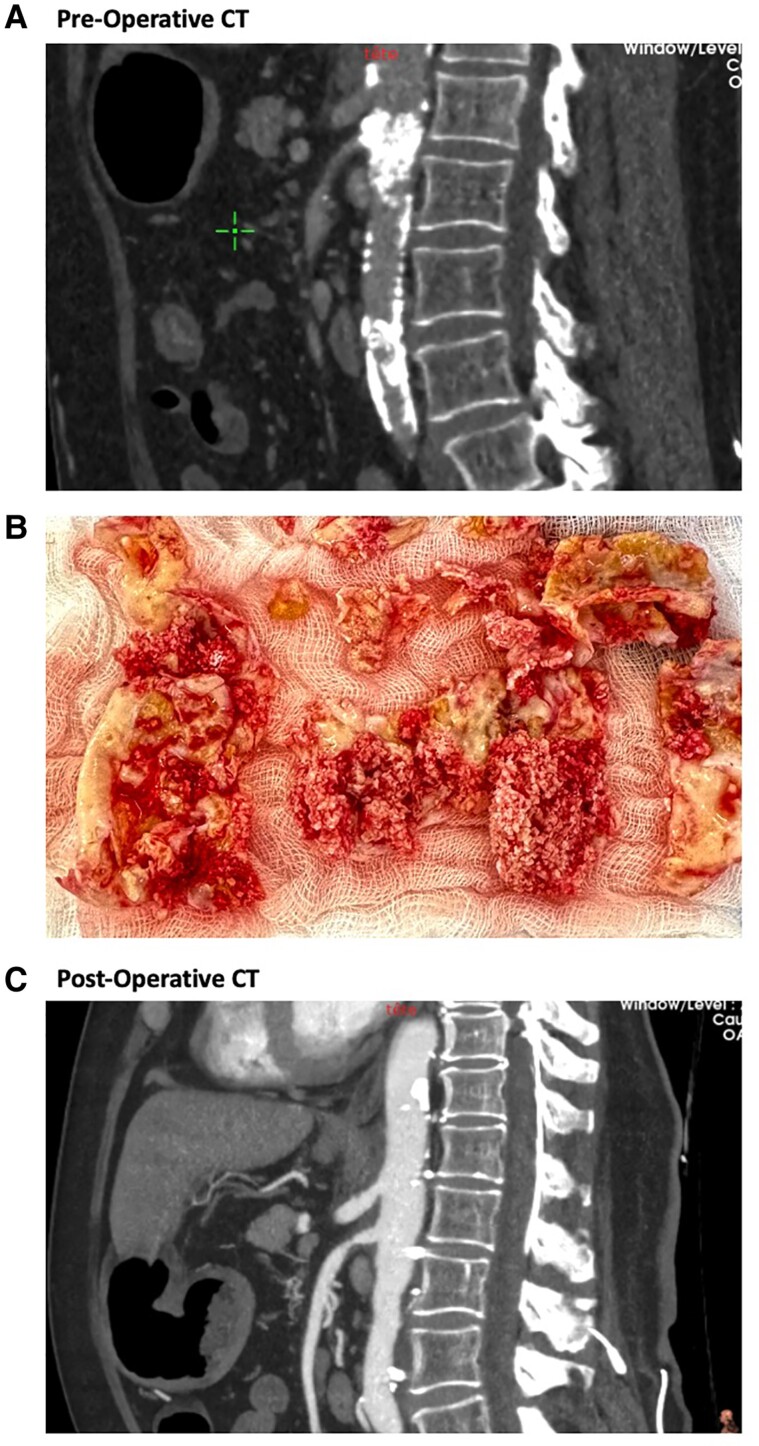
Representative computed tomography angiography images of a patient with coral reef on the abdominal aorta, before (*A*) and after (*C*) surgery as well as (*B*) intraoperative findings.
